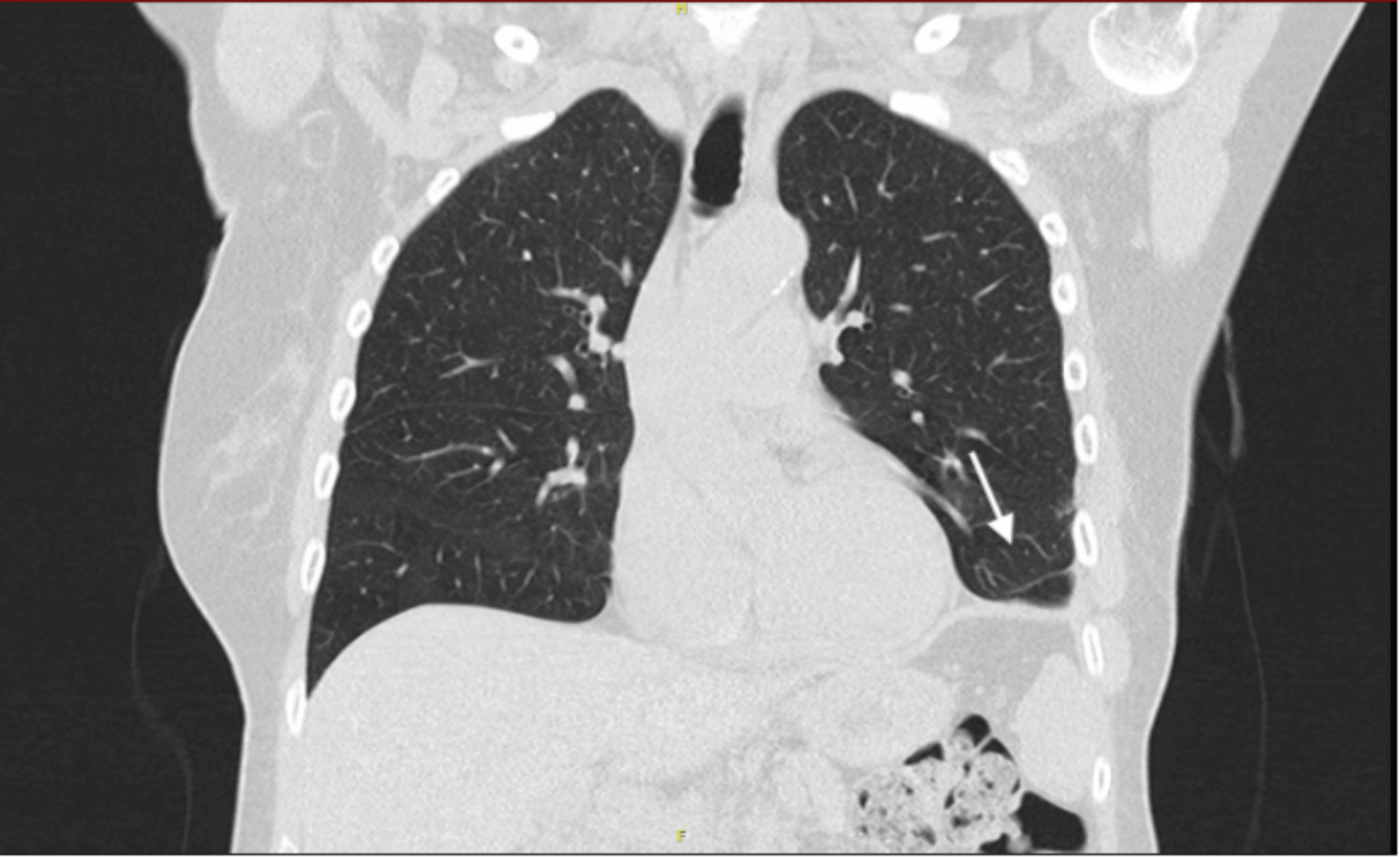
CT of the chest showing complete resection of the mass. Coronal plane, lung window.
